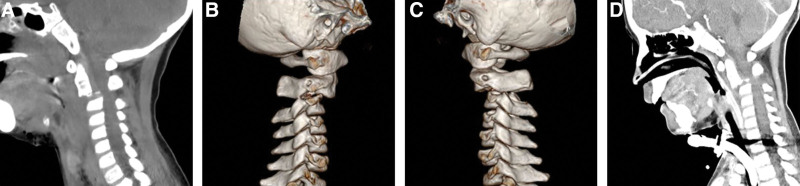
Three-dimensional CT (A, B and C) shows the fractures of the lower margin and IV° anterior spondylization of the C2 vertebral body, the fractures of the upper margin of the C3 vertebra and right transverse process, and left C2–C3 facet locking, while (D) shows no significant reduction in the C2 fracture-dislocation after 2 weeks of traction.
